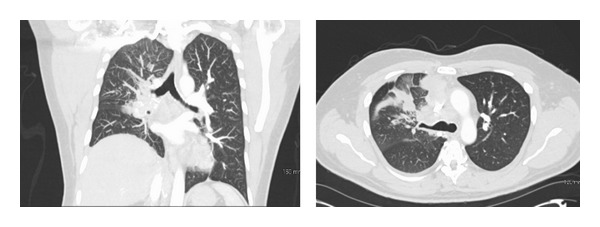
CT angiogram chest showing a large right mediastinal mass (8.8 × 6.2 × 9.6 cm), consistent with malignancy involving the right cardiac atrium with occlusion of the right main pulmonary artery which is either compressed or invaded by the mass, and nonopacification of right pulmonary arteries. No left pulmonary embolus is seen. Metastatic adenopathy in the mediastinum and probably right hilum. There are enlarged mediastinal lymph nodes, measuring up to 2.6 × 2.2 cm in the precarinal region and 2.2 × 1.9 cm in the anterior superior mediastinum.
